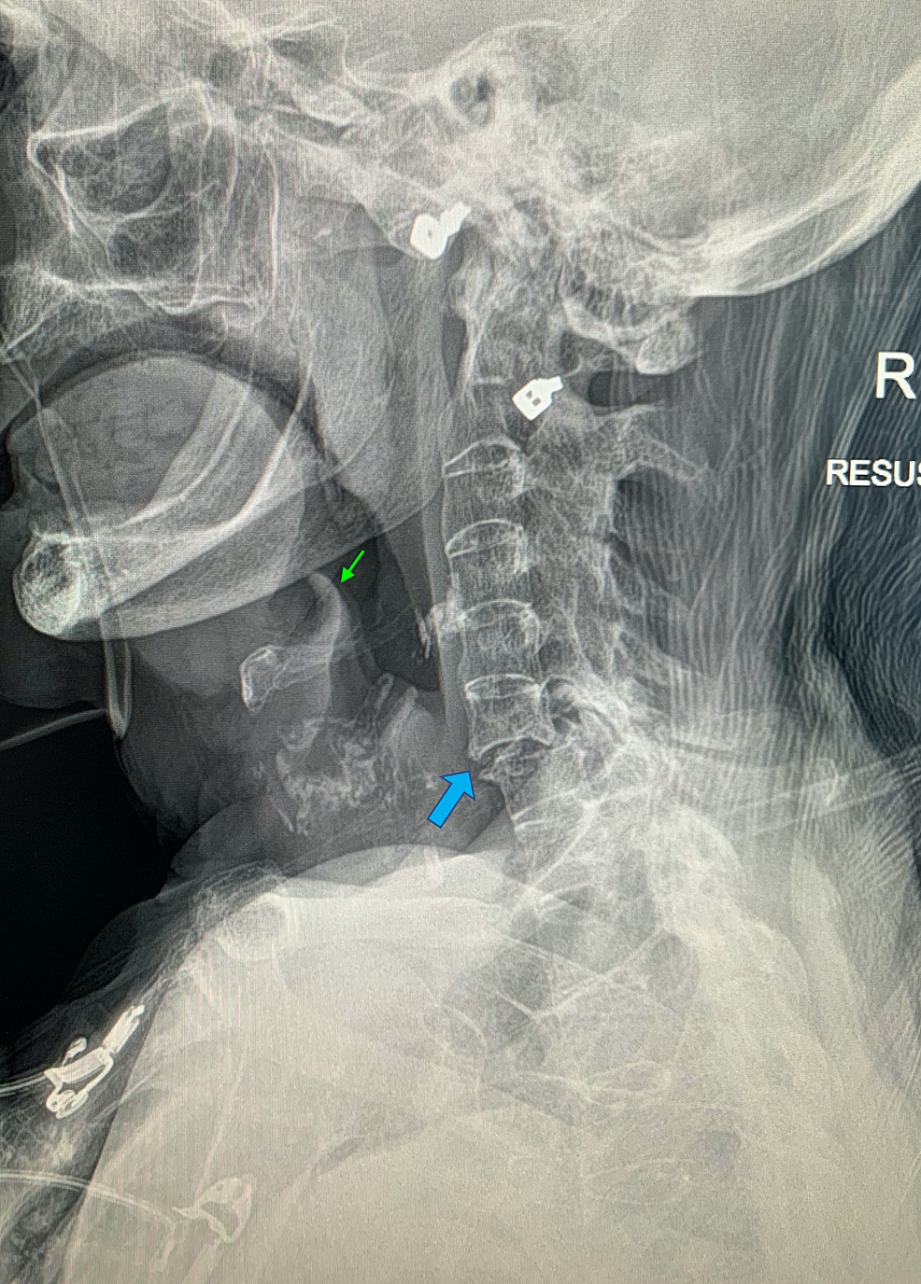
Radiograph of the lateral neck. The image demonstrates normal thickness of the epiglottis (green arrow) with no effacement of the vallecula, degenerative cervical spine secondary to ankylosing spondylitis, fusion of cervical vertebrae C2-C6, and grade 1 anterolisthesis of C6 on C7 (blue arrow).
